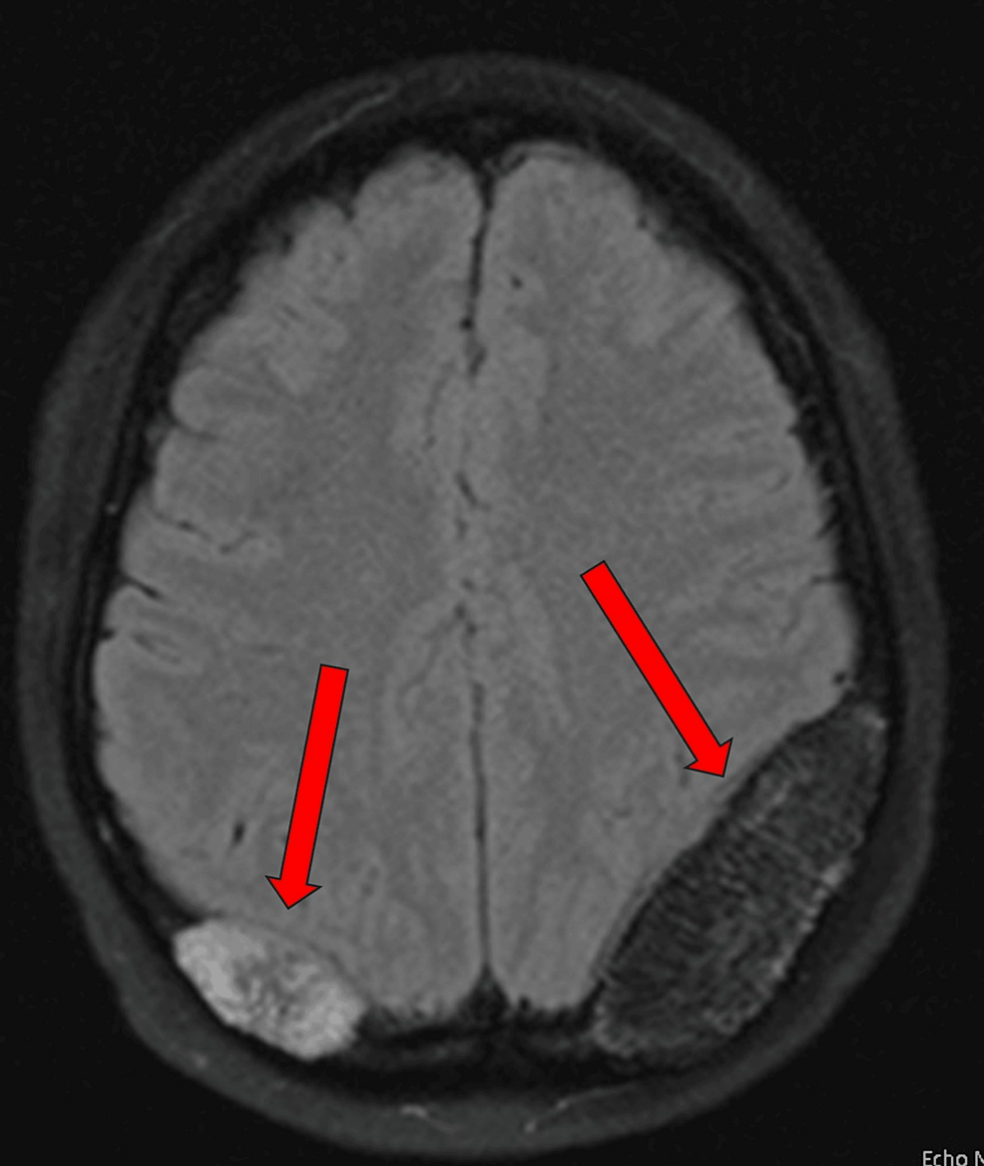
MRI brain T2 axial flair This is the follow-up imaging for the patient's brain masses found on MRI, showing growth of the right-sided lesion with the stable left-side lesion. MRI, magnetic resonance imaging
